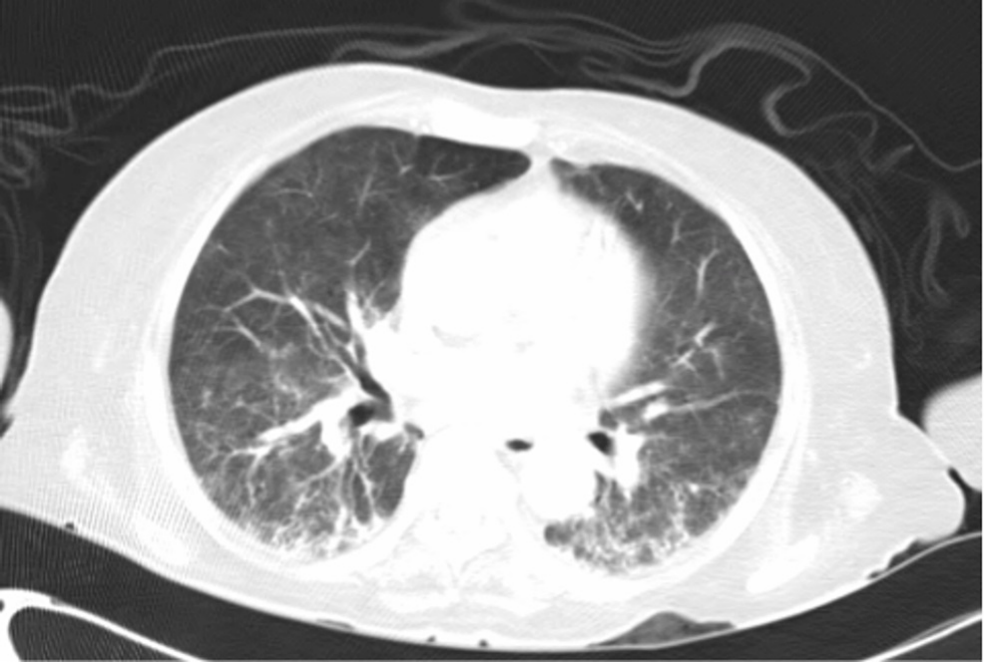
Repeat computed tomography of the chest
